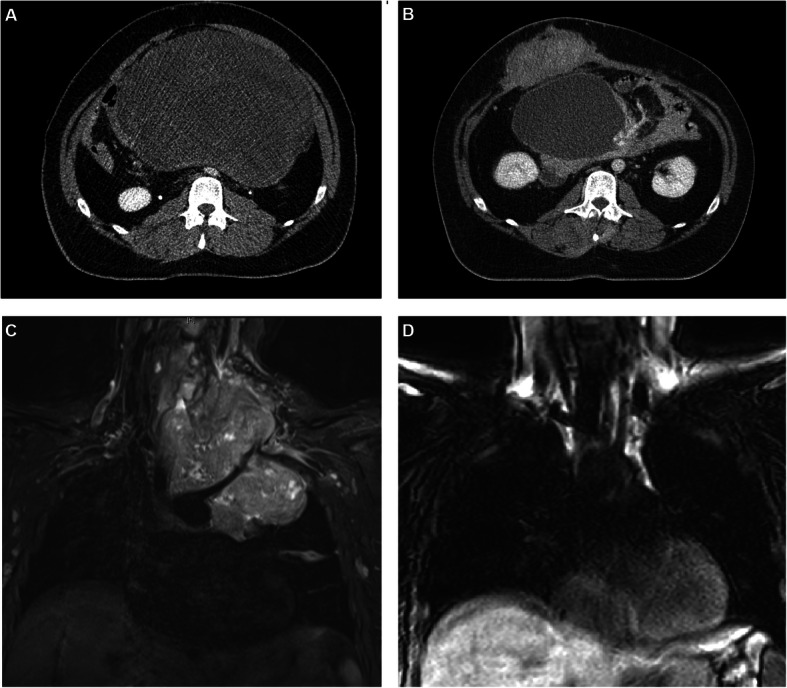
Examples of patients with desmoid tumors: A 27-year-old patient with a progressive, gigantic, FAP-associated mesenteric desmoid tumor, treatment was performed with photon-IMRT up to 50.4 Gy in 28 fractions, B: The patient developed a new symptomatic desmoid tumor of the abdominal wall 7 years after the primary treatment and was treated with protons 56 Gy (RBE) in 28 fractions. The mesenteric desmoid regressed in size, and stabilized with a cystic appearance on CT and MRI (not shown). C: Thirty-two-year-old patient who was pregnant at diagnosis of a gigantic cervico-thoracic desmoid tumor. The patient was treated beginning in the 28th week of pregnancy with protons, however the tumor progressed rapidly and the treatment was discontinued at 30 Gy (RBE). Caesarean section was performed and treatment with methotrexate and vinorelbine initiated. The tumor regress significantly after that
